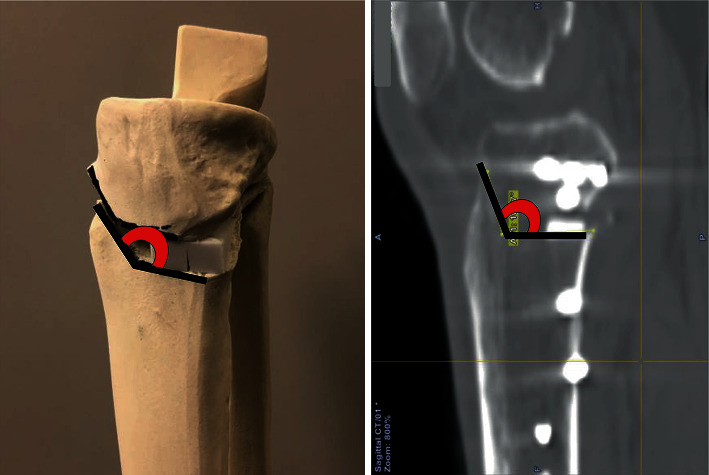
Measurement of the flange angle. We measured the angle between the ascending and transverse osteotomy lines on the anterior osteotomised surface where the flange was formed with the distal tibial osteotomised fragment (this study defined it so that sagittal plane on the CT could pass through the most anterior point of the tibial tuberosity).
